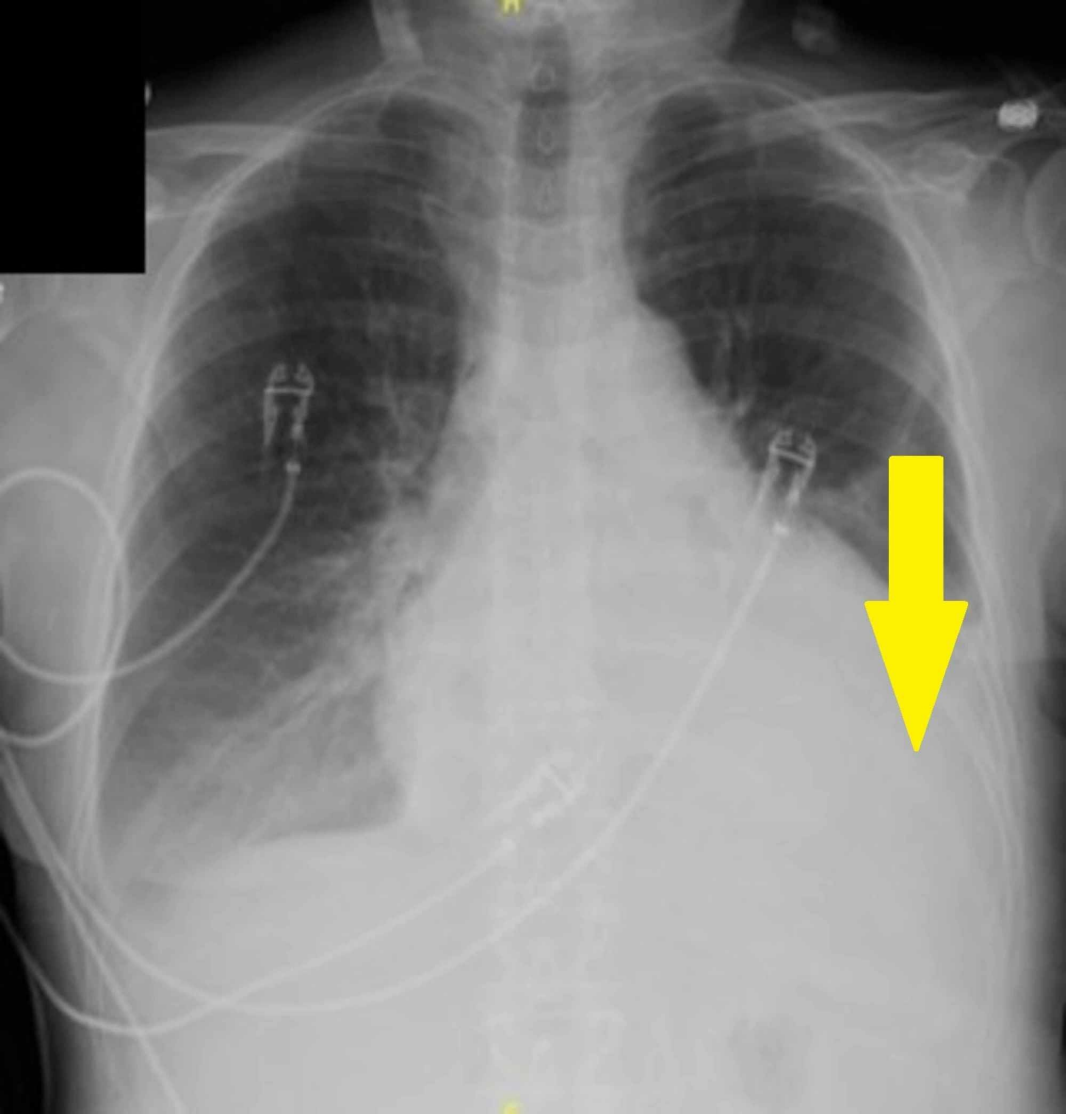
Chest Radiograph - Pleural Effusion
